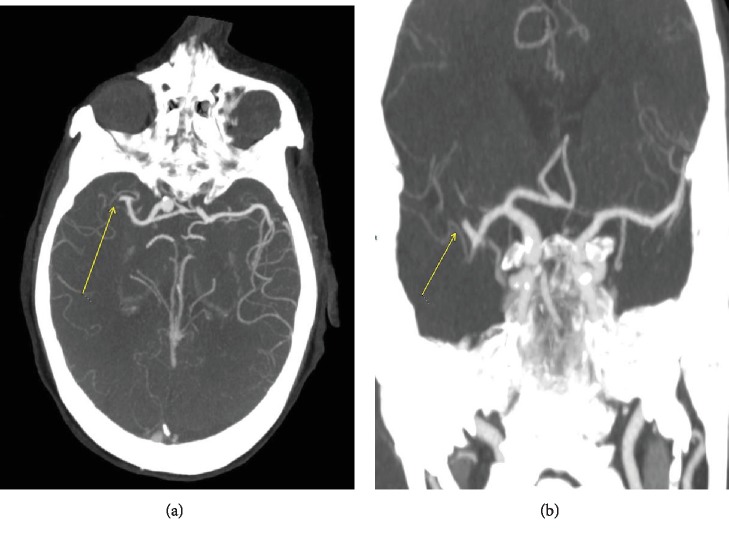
(a, b) CT angiogram of the head shows abrupt occlusion of the branch of the middle cerebral artery (arrows).
